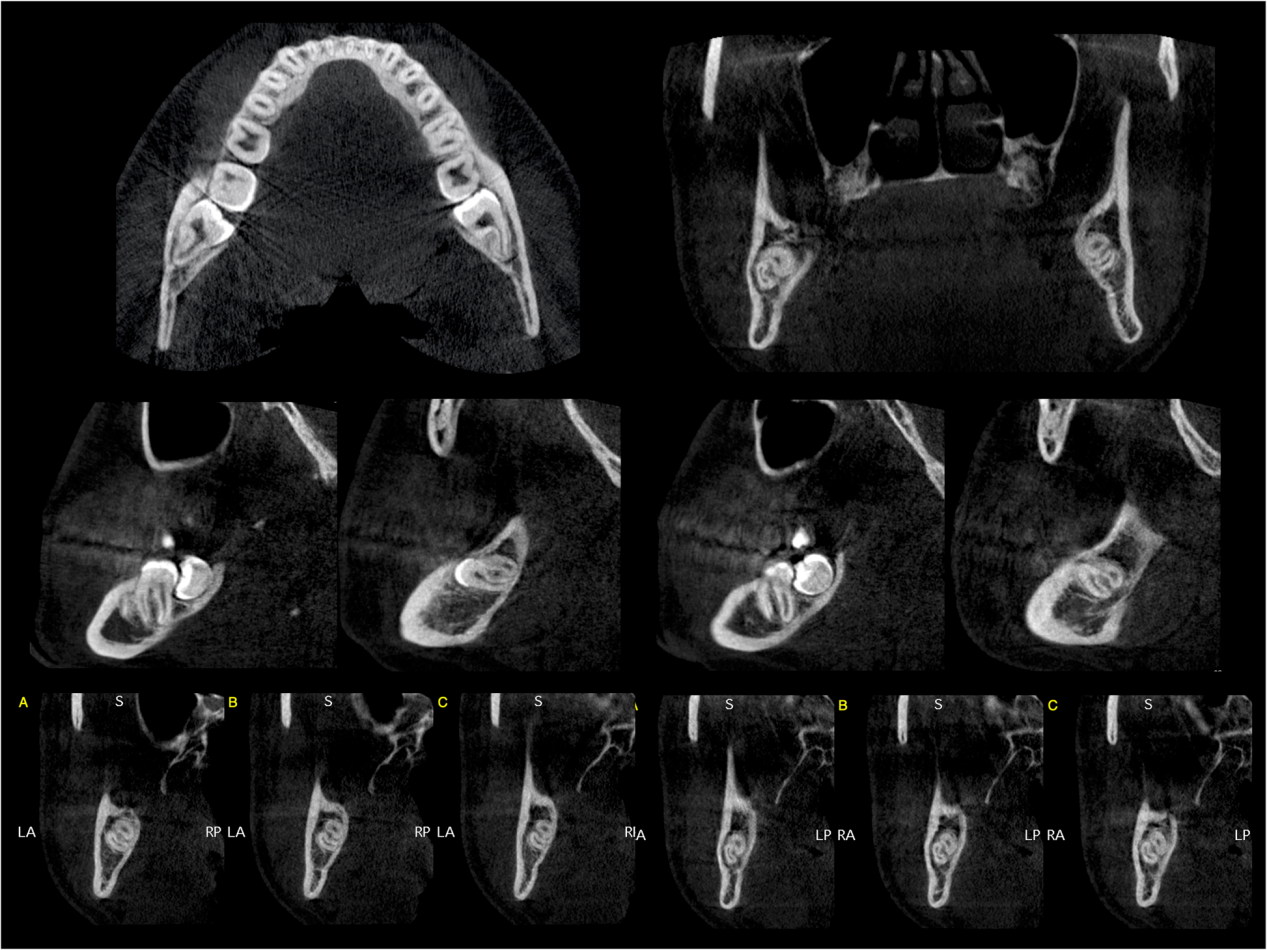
A pre-surgical evaluation with CBCT scan was performed to assess the presumptive surgical risk for each M3M
